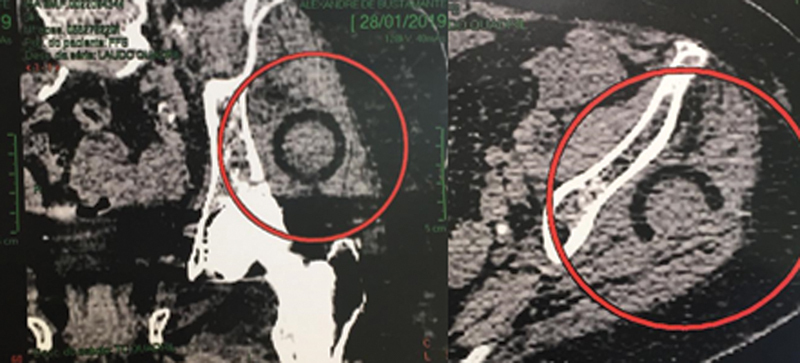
(A) Coronal section of computed tomography. The red circle shows polyethylene component in soft tissues, (B) In the axial cut, a red circle evidencing polyethylene.
